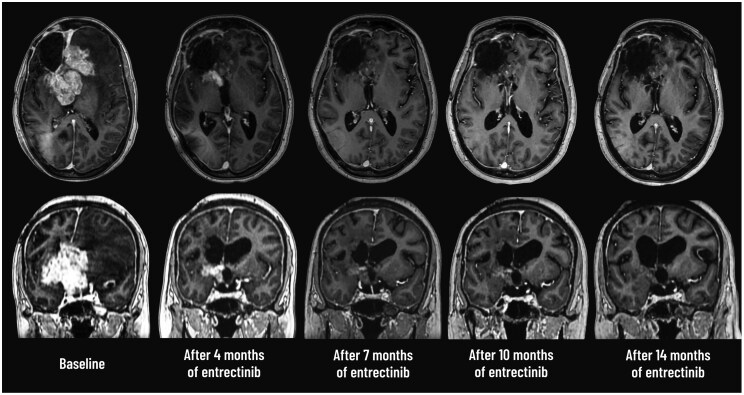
Radiological evolution of the tumor under entrectinib therapy. Axial, and coronal post-contrast T1-weighted MRI sequences obtained at baseline, and after 4, 7, 10 and 14 months of entrectinib treatment (from left to right). A marked and progressive reduction in the volume of the enhancing tumor is observed over time, indicating a sustained radiological response.
